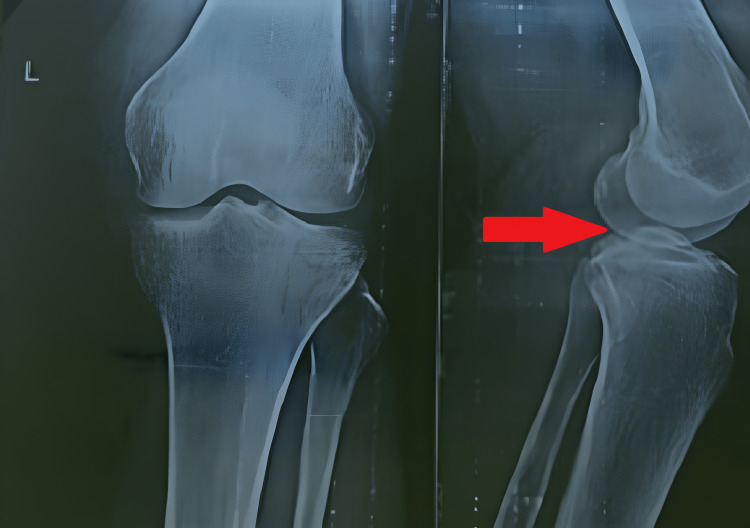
Left knee X-ray revealing injury to the posterior tibial plateau (red arrow)
